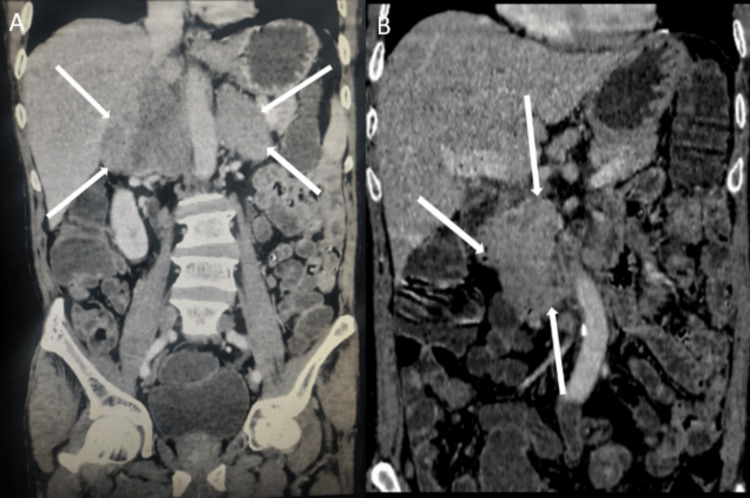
A: Coronal section of contrast-enhanced CT of the abdomen and pelvis showing bilateral adrenal metastasis. B: Coronal section of contrast-enhanced CT showing a hypodense omental metastatic mass in the subhepatic area.
